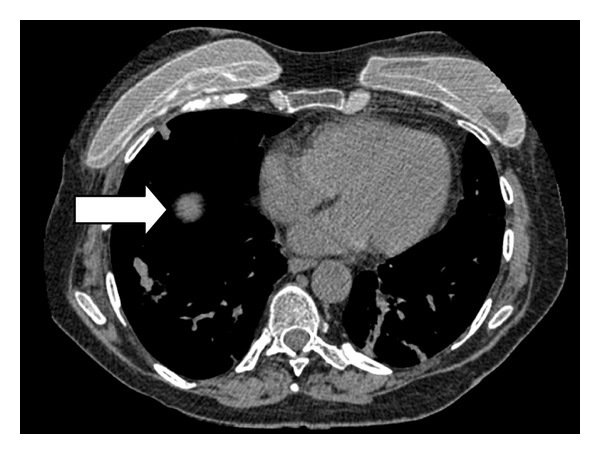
CT chest showing right lower lobe pulmonary nodule (arrow).
